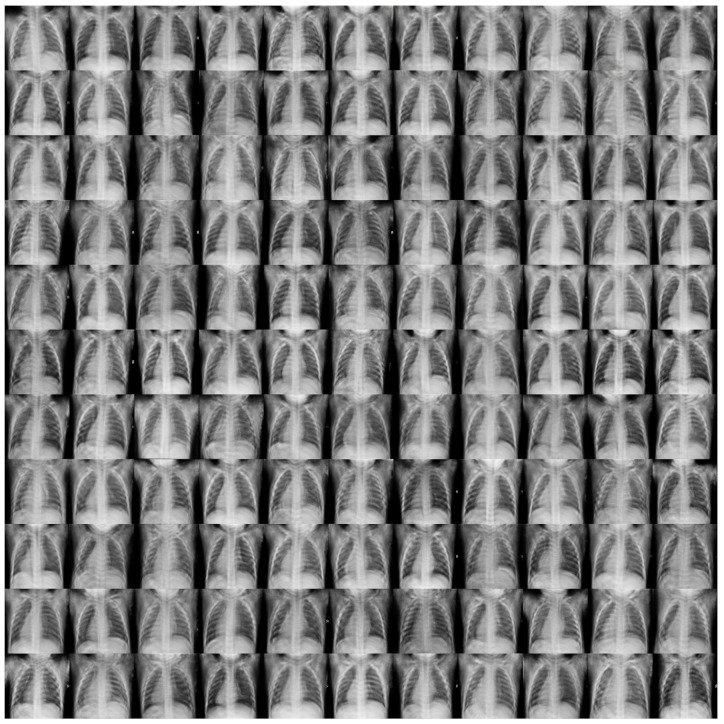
Unbiased, random samples from our chest X-ray generation model, which underwent continuous-time training and used T = 20,000 during image creation. The model’s parameters and hyperparameters are fine-tuned to enhance the quality of the synthesized images, ensuring the generated medical images accurately represent the true anatomical and physiological features.
